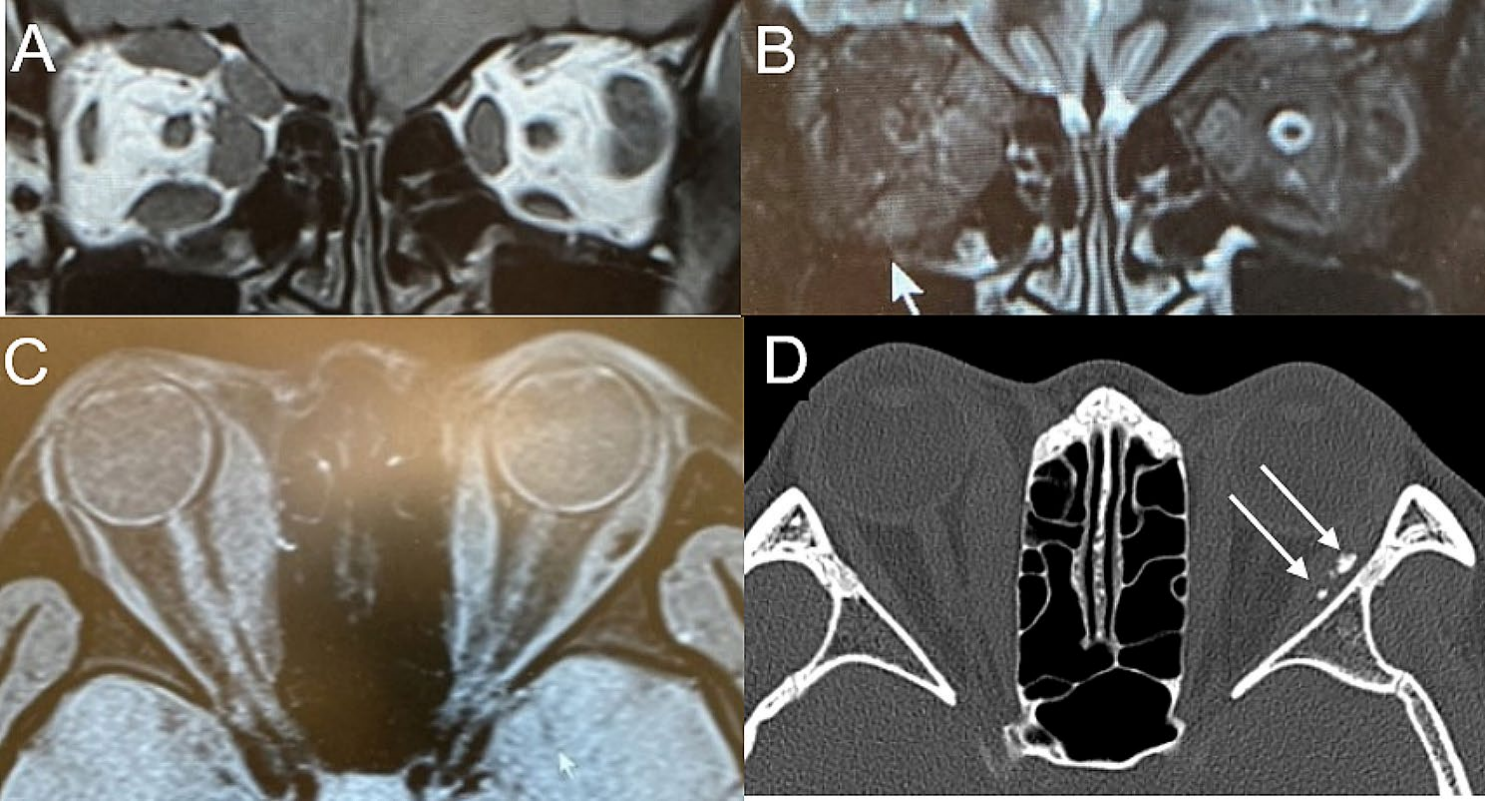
(A) Coronal T1-weighted MRI image depicting bilateral extraocular muscle thickening with tendon sparing (B) Coronal orbital image using Short-Tau Inversion-Recovery (STIR) sequence, highlighting inhomogeneous enhancement in the external muscles of both eyes (C) Axial T1-weighted MRI image displaying enlargement of bilateral medial rectus muscles and left lateral rectus (D) CT scan revealing calcification (arrow) within the right lateral rectus muscle
